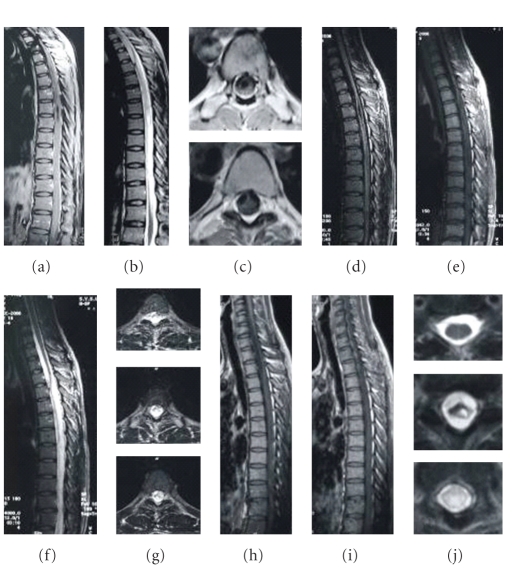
A 25-year-old woman was admitted due to the progressive weakness and parasthesia in both legs with voiding problem for 3 months. After 2-year ATT, her situation improved with good recovery of spinal function. The MRI changes are as follows: (a) first MRI performed in August 2005, T1 weighted image showing cord edema from T1 to bottom in the sagittal plane, with focal atrophy and syrinx cavity, (b) T2-weighted image showing a contiguous area of increased signal intensity, (c) cavity and band-like structure on axial T1-weighted images, (d, e) after one year of treatment, the swelling of cervical spinal cord greatly dissolved on sagittal T1-weighted image; the syrinx cavity is obviously diminished, (f) contiguous abnormal signal still left but diminished on the sagittal T2-weighted image, (g) the lesion is still obvious on axial T2-weighted images, (h, i) three years after treatment, the syrinx cavity is not obvious, and (j) some parts of thoracic cord restore normal signal on T2-weighted axial cord images. A small cavity is visible on T8, and increased signal intensity still exists below T9.
